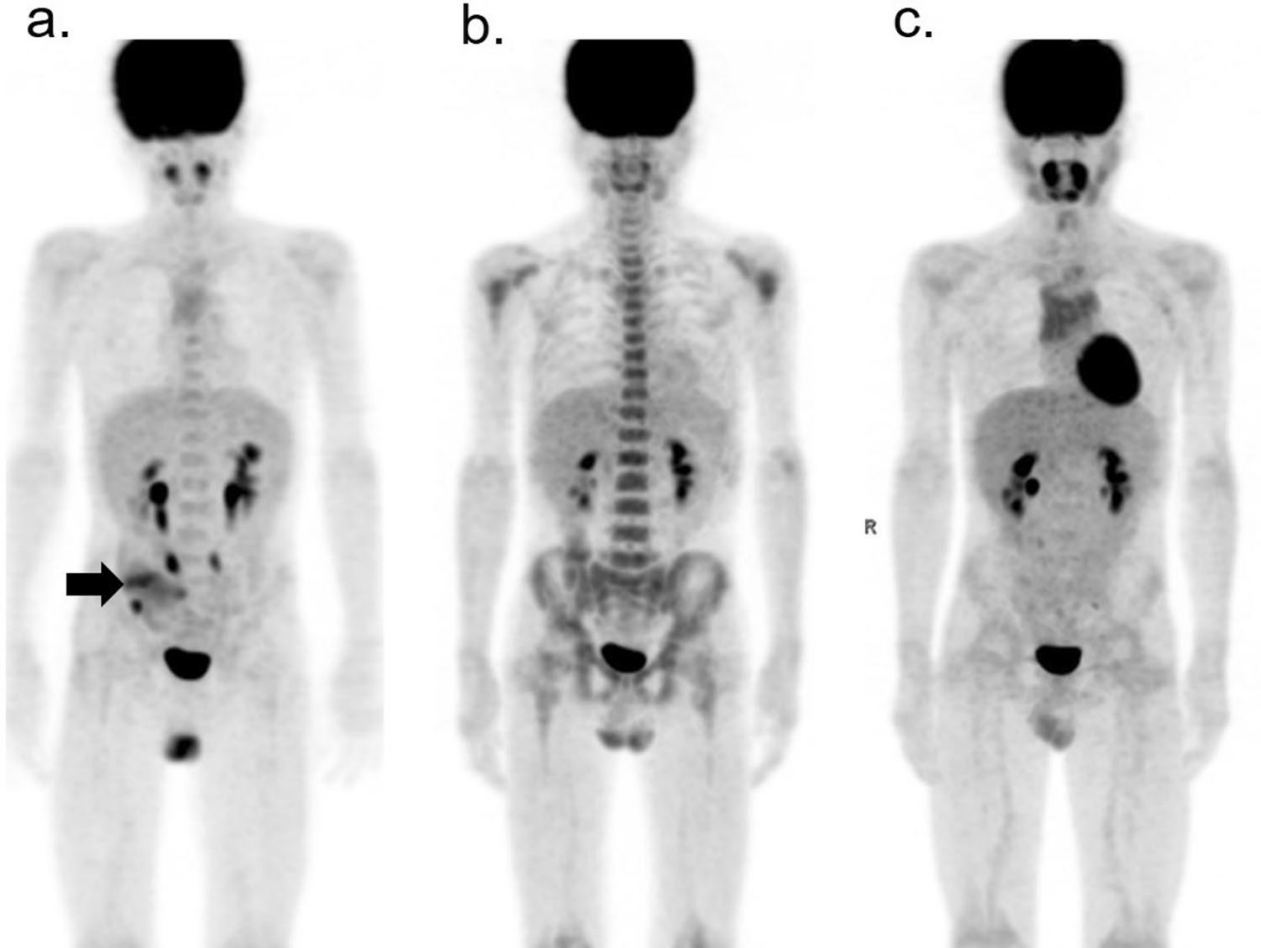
Serial changes in physiological FDG uptake in a pediatric patient with lymphoma: a before treatment (arrow shows the primary spot), b 1 month after the end of chemotherapy, and c 1 year after treatment. The image b shows diffusely increased uptake in the bone marrow. One year after chemotherapy, rebound uptake is seen in the thymus and tonsils, both of which seem to be enlarged. The bone marrow uptake has returned to its initial levels
